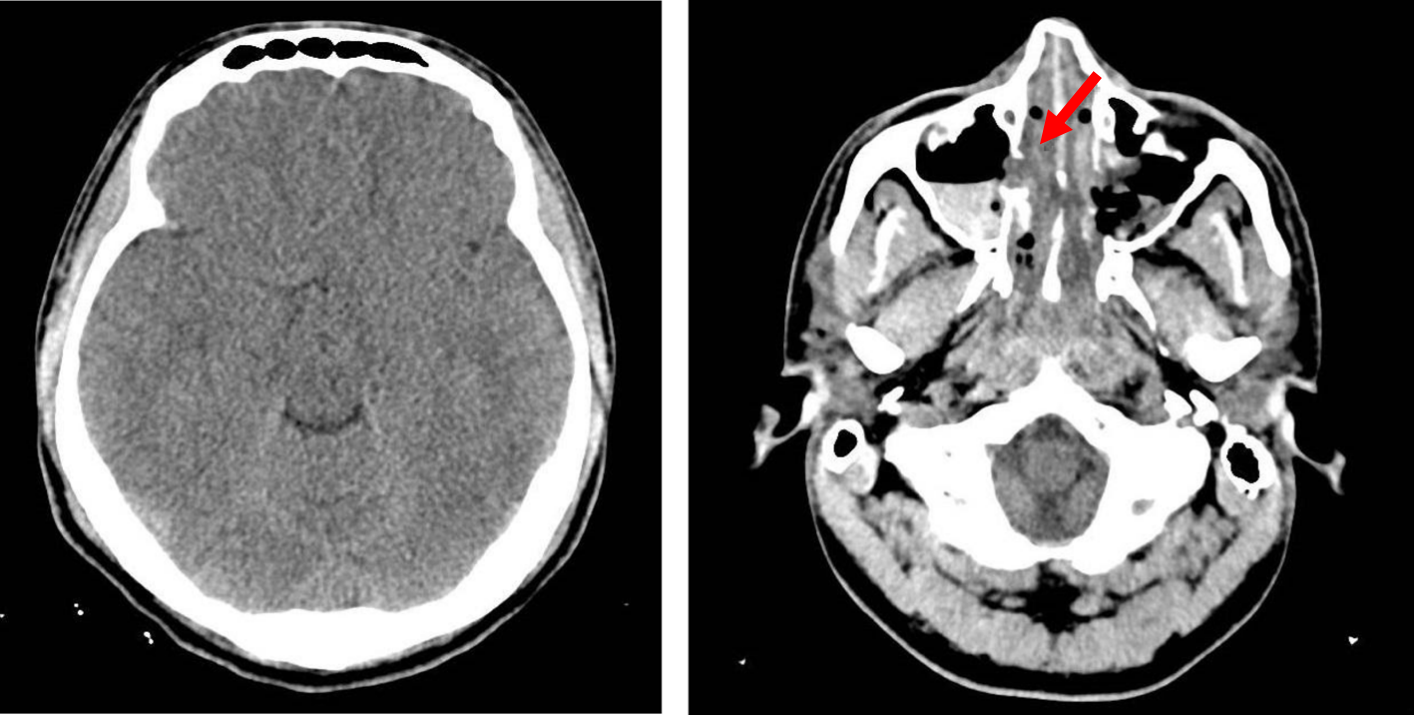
Head CT at admission shown Global Edema (Left-sided) and multiple Maxillary Sinuses fractures (Right-sided red arrow)
